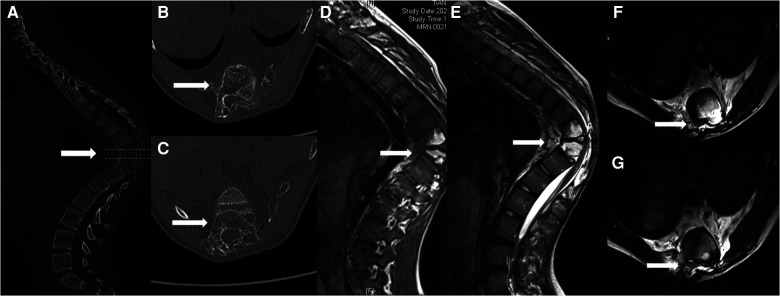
(A) Computed tomography images of the thoracic vertebrae indicate marked osteolysis within the T10/T11 vertebrae (arrows); (B) Osteolysis within the T10; (C) Osteolysis within the T11; (D) High-signal intensity was observed on the T1-weighted image within the T10/11 vertebrae (arrows); (E) High-signal intensity was observed on the T2-weighted image within the T10/11 vertebrae (arrows); (F) spinal cord compression was observed on the T2-weighted image within the T10 level (arrows); (G) spinal cord compression was observed on the T2-weighted image within the T11 level (arrows).
